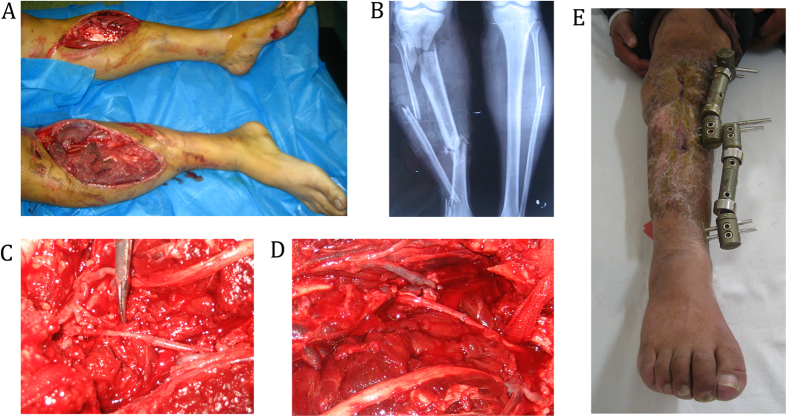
A 25-year-old man sustained open injuries to both lower extremities, with ischemia that had increased over a 10-hour period. (A) Preoperative detection found injuries to the anteroposterior tibial and peroneal artery. (B) X-ray show the fracture in tibial and peroneal.(C,D) The far-end peroneal artery was bridged to the popliteal artery bifurcation using RSVG, and the near-end peroneal artery was anastomosed with the distal tibial artery. (E) the salvage of the limb was deemed successful, and the Fugl-Meyer upper-extremity score was 89 points.
